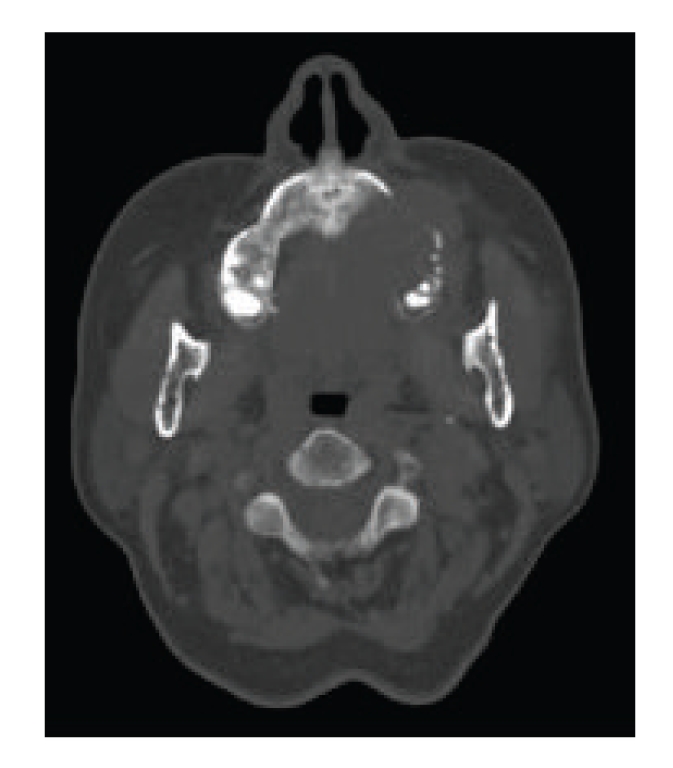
Plasmacytoma: axial noncontrast CT image at the level of left maxillary sinus demonstrates an expansile, destructive intraosseous lesion involving the left maxillary sinus.
